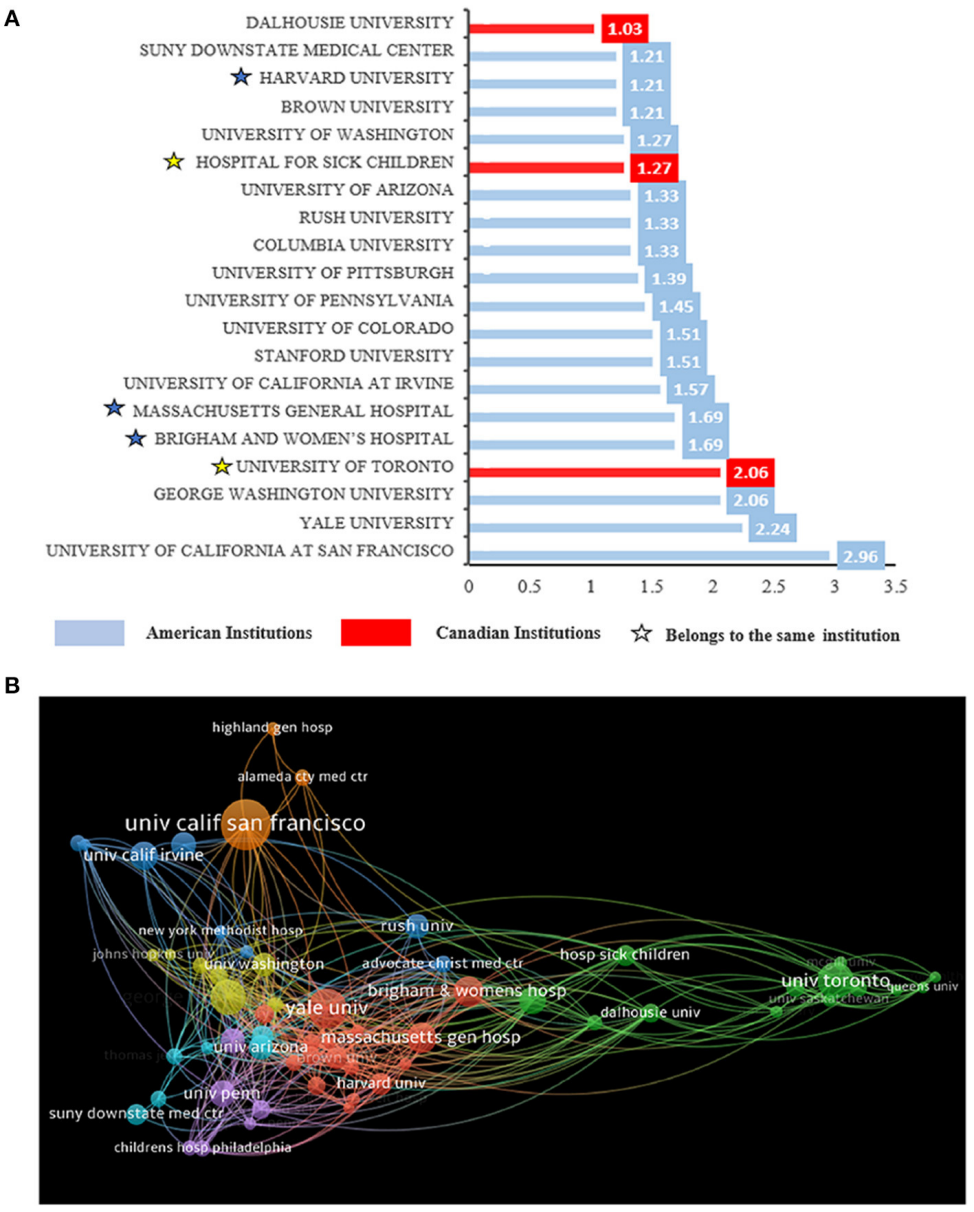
The distribution of institutions engaged in research on the use of ultrasound in emergency medicine. (A) Top 20 institutions by number of publications. Numbers represent the percentage of publications, with blue representing institutions in the United States and red representing institutions in Canada. Asterisks of the same color indicate that such organizations belong to a same organization. (B) The network of institutions produced in VOSviewer. The size of circles reveals the number of publications.
